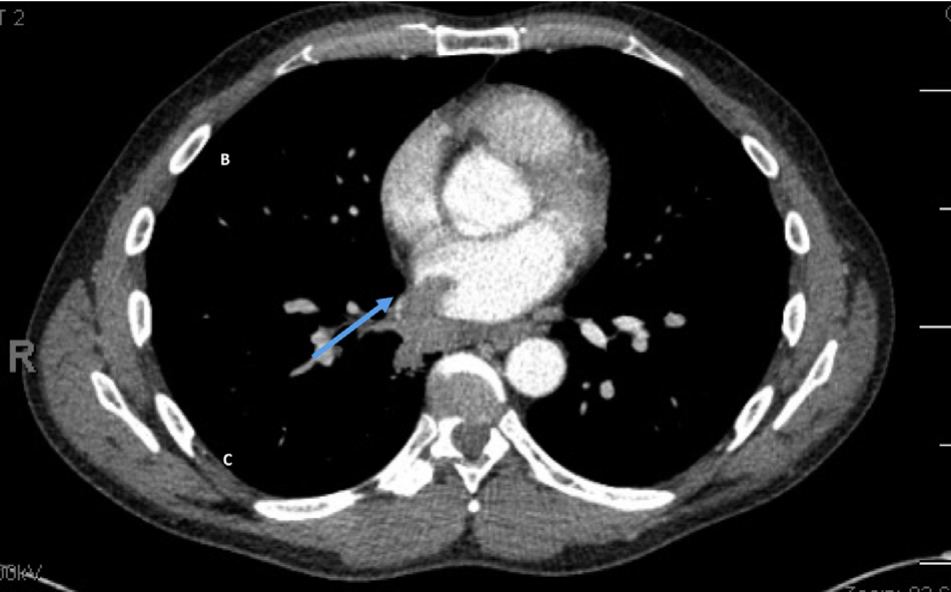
Contrast-enhanced CT scan demonstrating a solid tissue mass originating from the superior segment of the right lower lobe measuring 20 × 16 mm that invades the right lower pulmonary vein extending into the LA *(blue arrow)*.
